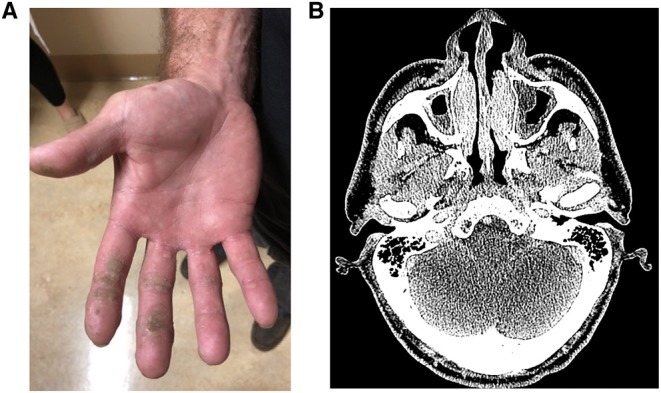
(A) Numerous, confluent cutaneous warts on palmar surface of hand. (B) CT sinuses showing turbinate edema and maxillary sinus mucosal thickening with layering fluid.
